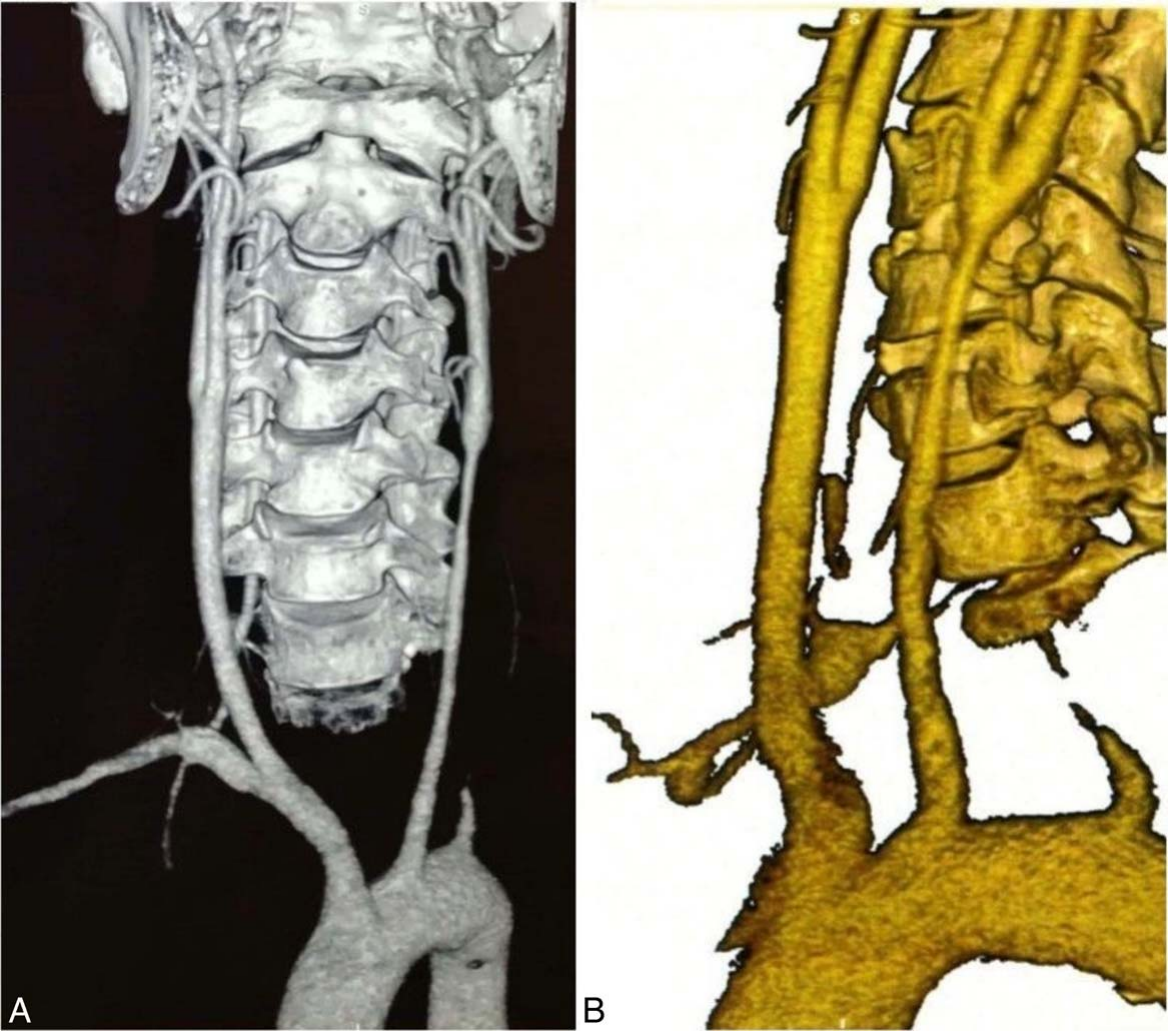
Computed tomography angiography demonstrating stenosis in the left common carotid artery, left vertebral artery, right subclavian artery, and occlusion of the left subclavian artery (A, B).
